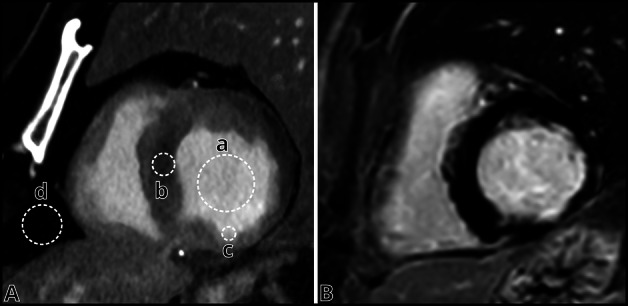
Region of interest measurements. Images from a 71-year-old man with a history of coronary artery disease. Mid-ventricular short axis late iodine enhancement (A) with corresponding late gadolinium enhancement from a previous cardiac MRI scan (B). Circular regions of interest indicate measurements for CT attenuation of the blood pool (HU_blood_, a), myocardium (HU_myocardium_, b) and late enhancing scar (HU_scar_, c), as well as image noise measured as the standard deviation of CT attenuation in fat (SD_fat_, d)
